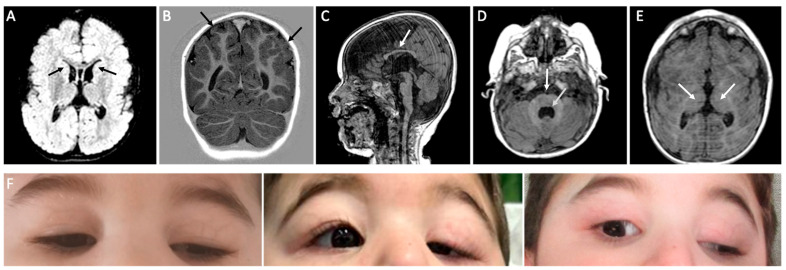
Brain MRI and clinical pictures of the proband. (A) Flair sequence, protrusion of the head of the caudate nuclei into the lateral ventricles giving the frontal horns a hooked configuration. (B) T1-w. Polymicrogyria-like cortical dysplasia predominantly at the fronto-parietal convexities bilaterally. (C) T1-w. Thin corpus callosum. (D) T1-w. Hypoplasia of the pons with central fissure and dilatation of the IV ventricle, dysmorphic appearance of cerebellar peduncles. (E) T1-w. Globular appearance of thalami. (F) Severe bilateral ptosis resulting from CFEOM is shown at different ages: 8 months (left, presurgical), 1.5 years (center, postsurgical, right eye), and 3.5 years (right, postsurgical right eye).
